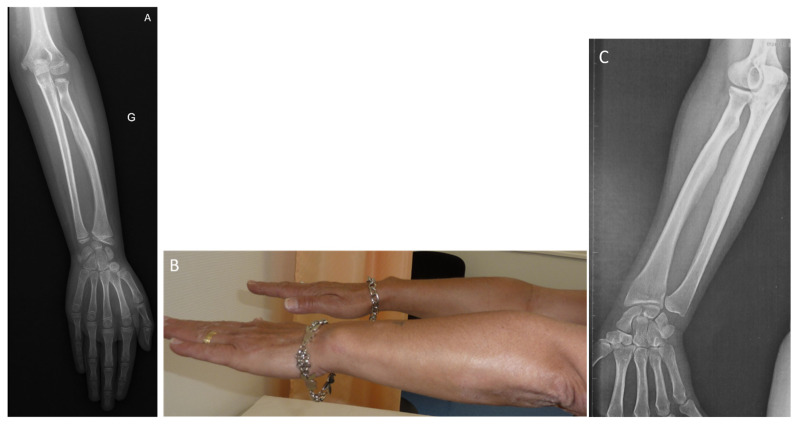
(A) Radiograph of the left forearm of individual IV.1 at 11 years and 7 months showing medial inclination of the distal radial epiphysis (Madelung deformity) as well as a triangularization of the carpus resulting from the inclination of the radial epiphysis. G is “left” in french (gauche). (B) Photograph of the forearms of individual III.2 (mother of individual IV.1) at 43 years old showing medial inclination of the distal radial epiphysis (Madelung deformity). (C) Radiograph of the right forearm of individual III.2 (mother of individual IV.1) at 43 years old showing medial inclination of the distal radial epiphysis (Madelung deformity).
